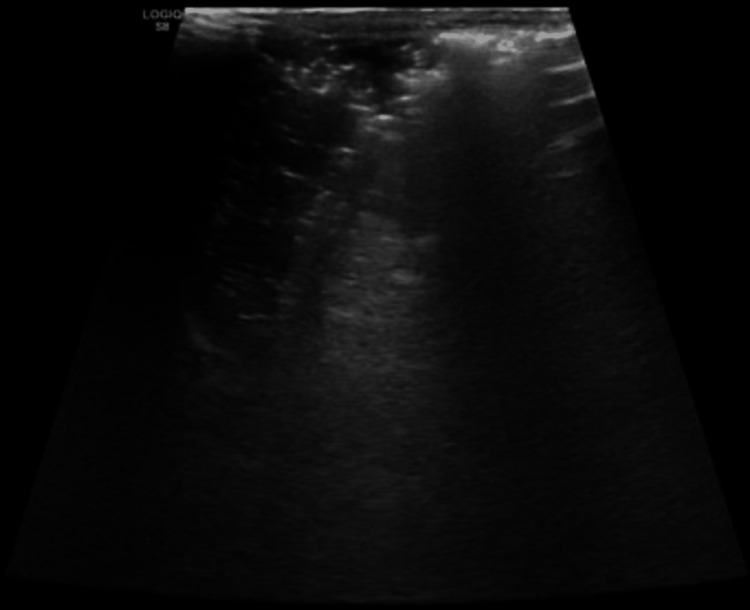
Abdominal ultrasound showing spleen normal in size with innumerable echogenic foci.
